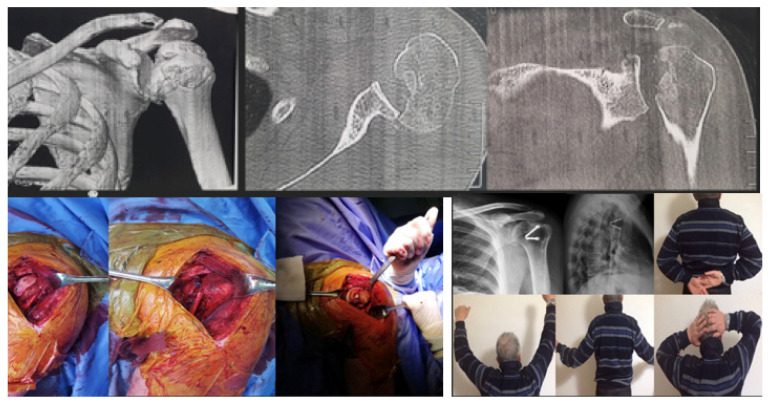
A 54-year-old man with type 1 injury 4 weeks after trauma. X-ray and clinical check-up at 12 months.
